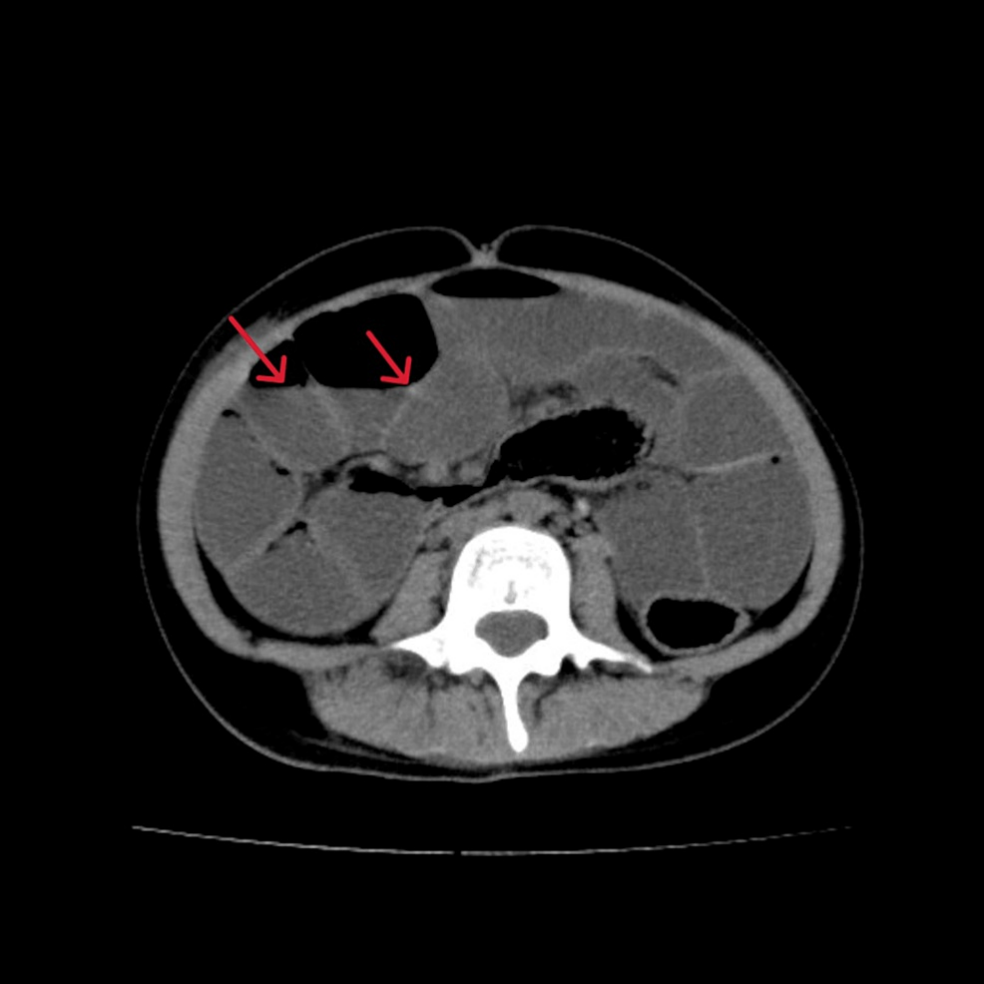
Plain CT of the abdomen shows no ascites, free air, intestinal axis torsion, or intestinal stenosis. Red arrows show niveau formation.
